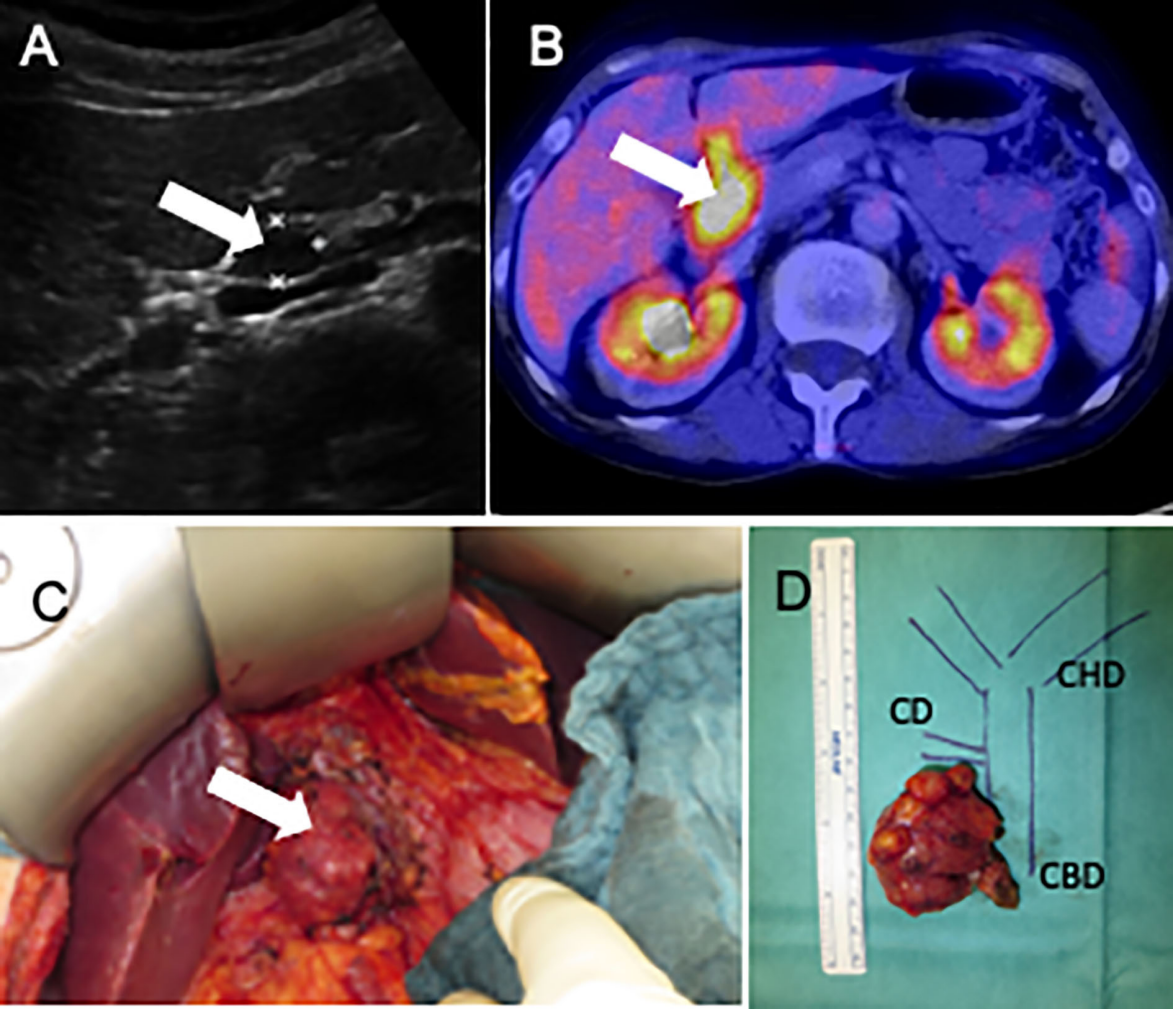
Abdominal ultrasonography (A) [18F]FDG-PET-CT (B), photography surgery 10/2018, Lymph node metastases *in situ*
